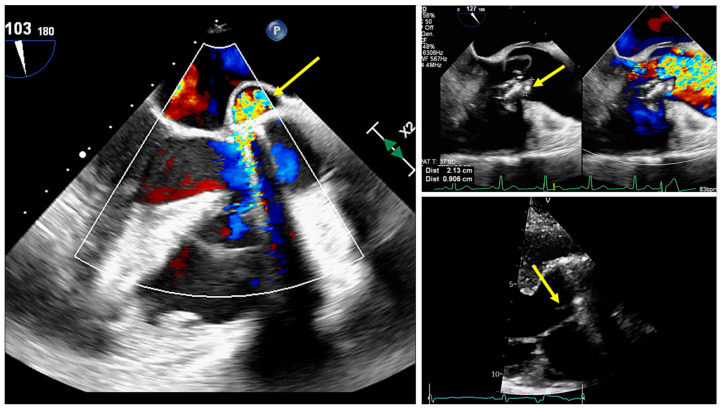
In clockwise order: transesophageal echocardiographic image of a pseudoaneurysm of mitral-aortic intervalvular fibrosa in a patient with prosthetic aortic valve and coarctation of aorta; transesophageal echocardiographic image of vegetation attached to a bicuspid aortic valve; transthoracic echocardiographic image of vegetation on prosthetic aortic valve. Yellow arrows point to pseudoaneurysm (image on the left) and to vegetations (images on the right).
